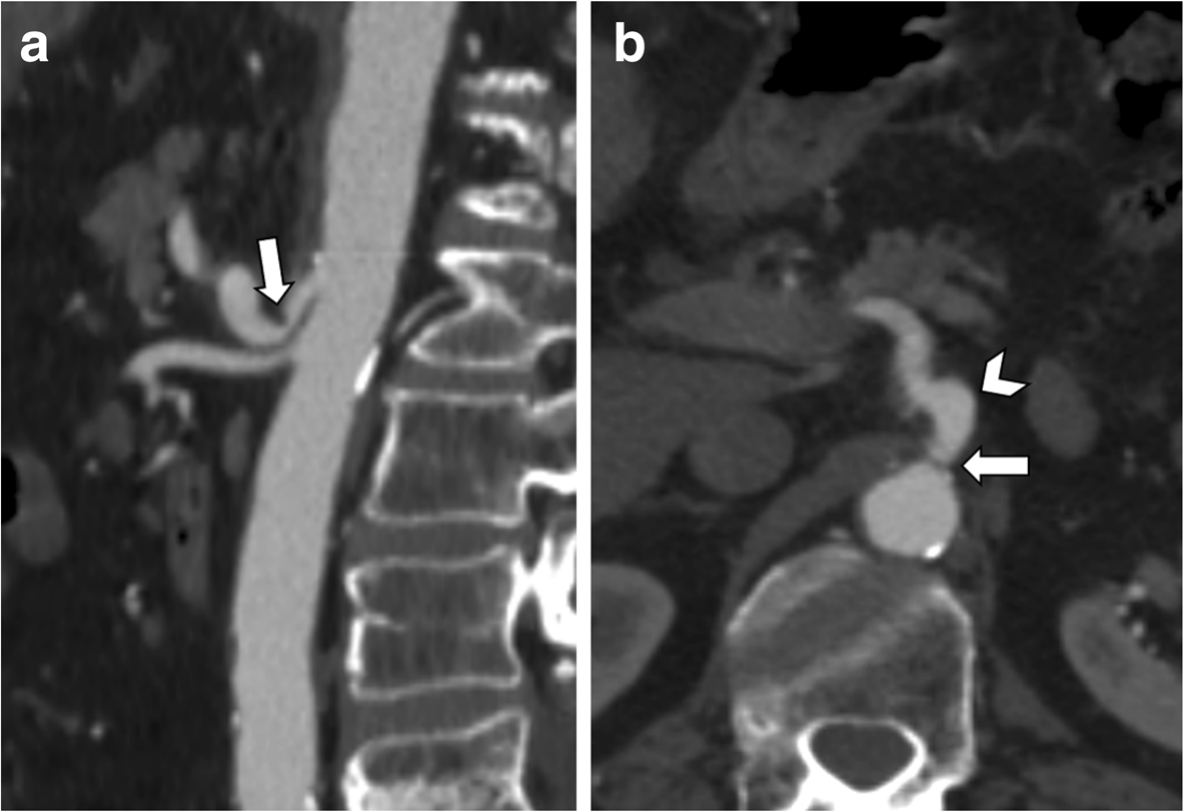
A 65-year-old man with median arcuate ligament compressing the celiac artery. a Sagittal CT image shows the “hooked appearance” (arrow) with severe stenosis at the origin of the celiac artery, in the absence of atherosclerotic plaques. b Corresponding axial CT image shows celiac artery compression (arrow) with post-stenotic dilatation (arrowhead)
